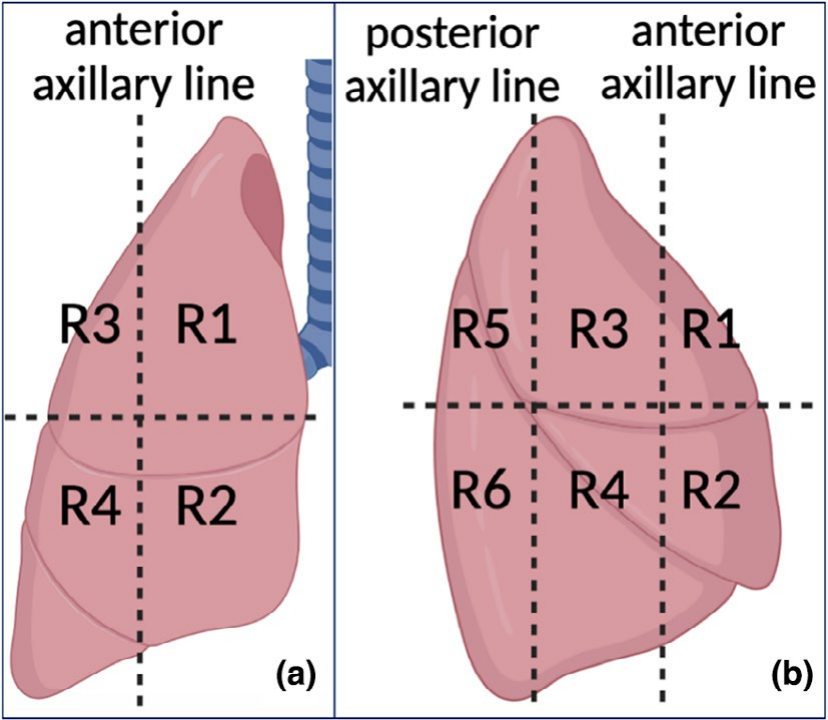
Regions for standardized lung ultrasound examination. For demonstration, the right lung is divided into anterior, lateral, and posterior regions based on anatomical landmarks (anterior and posterior axillary lines and internipple line). (a) Anterior view of the lung. (b) Lateral view of the lung. The regions are defined as follows: R1: Region 1, anterior superior; R2: Region 2, anterior inferior; R3: Region 3, lateral superior; R4: Region 4, lateral inferior; R5: Region 5, posterior superior; R6: Region 6, posterior inferior. Created in BioRender.
